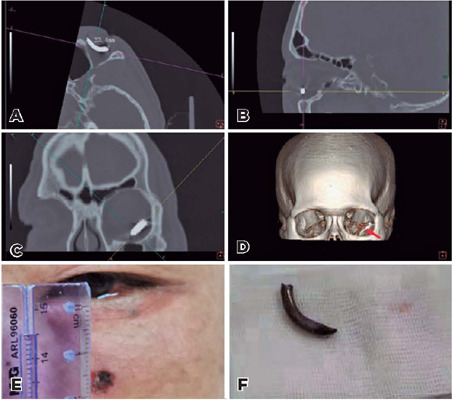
Axial (A), sagittal (B), and coronal computed tomography (CT) images (C)
